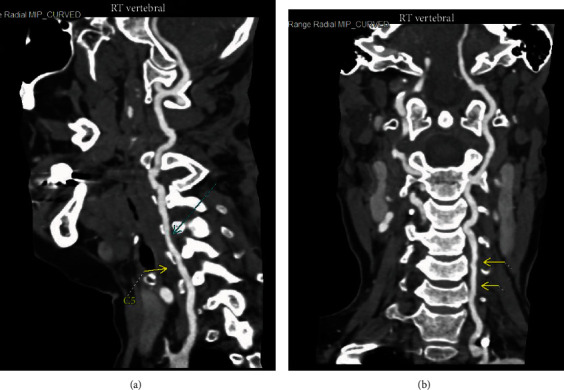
(a) Computed tomography angiography imaging showing the right vertebral dissection (cyan arrow). (b) Computed tomography angiography imaging showing the left vertebral artery dissection (yellow arrows).
